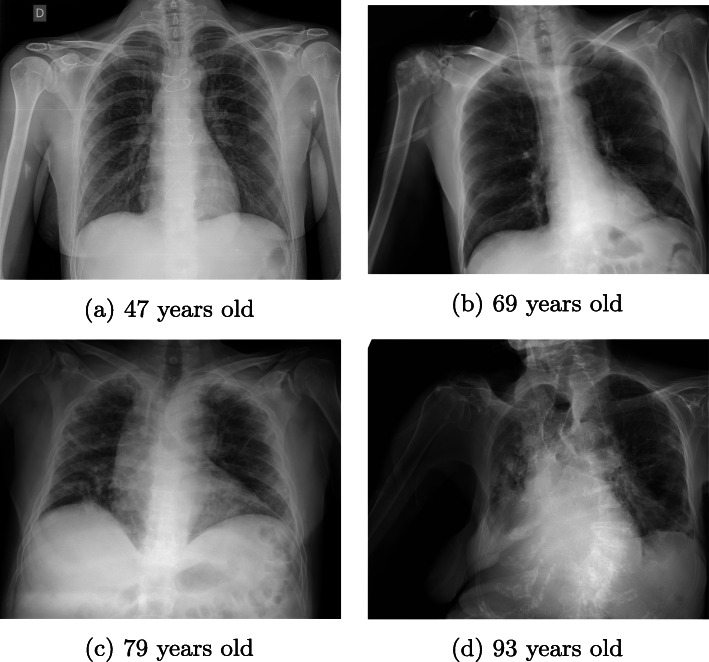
Example of four representative chest X-ray images of patients of different ages diagnosed with COVID-19
